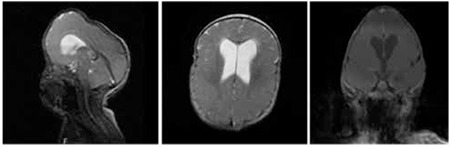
Patient’s magnetic resonance imaging: presence of hydrocephalus and hypoplasia of corpus callosum and inferior vermis
